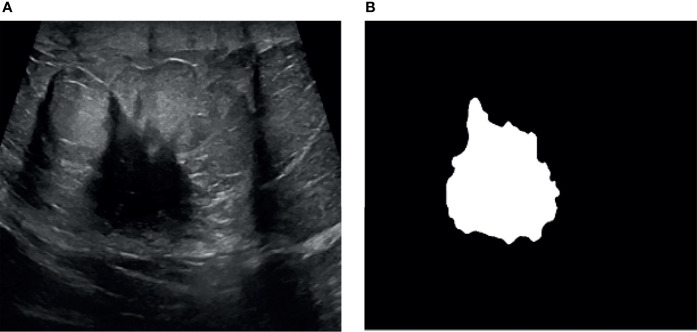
ROI segmentation. (A) Ultrasound image and (B) segmented image.
